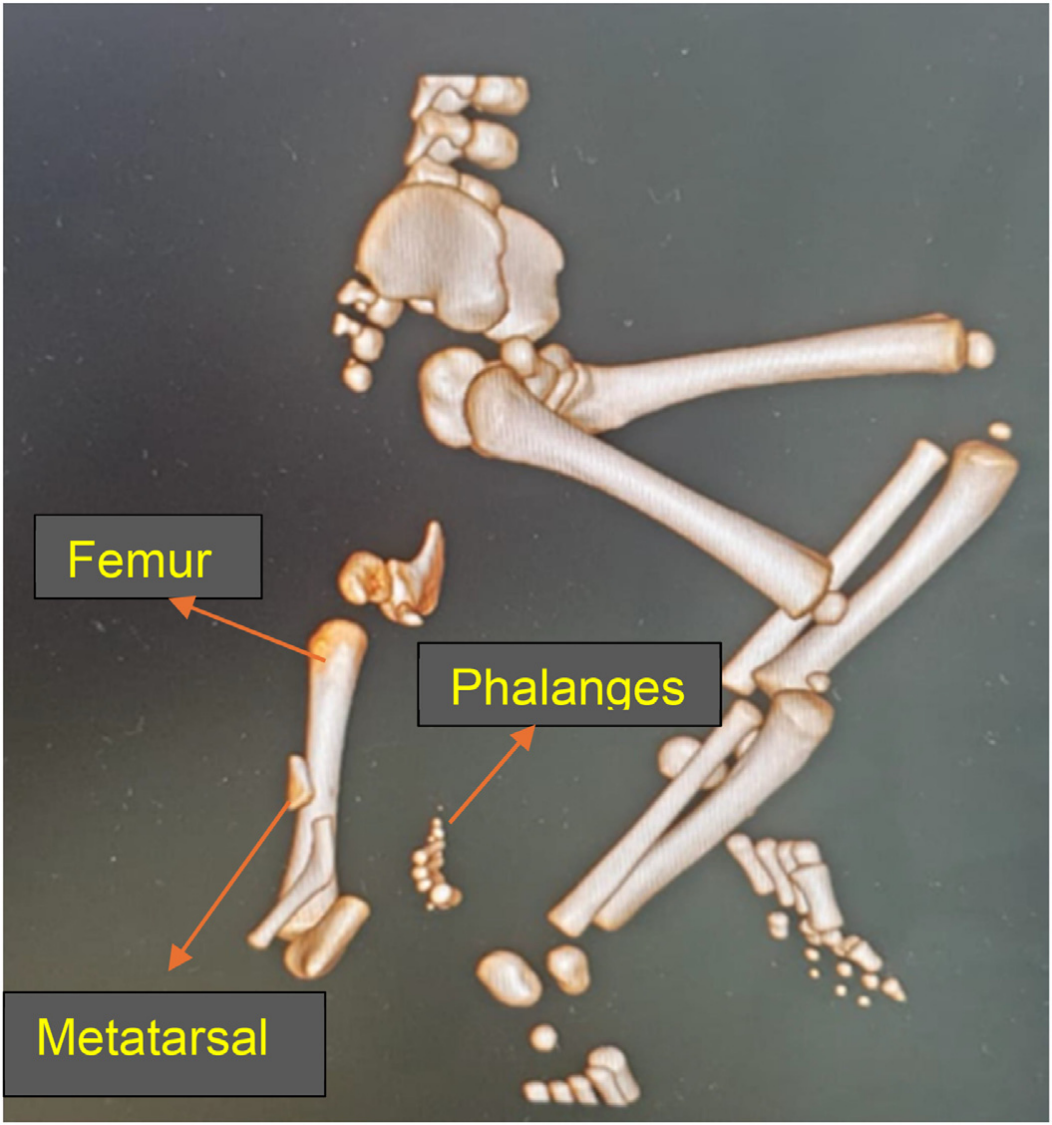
CT image of Extra-limb showing bony elements with fully developed femur and proximal articulation along the partly formed acetabulum. Metatarsal and phalanges of extra-feet also could be seen.
